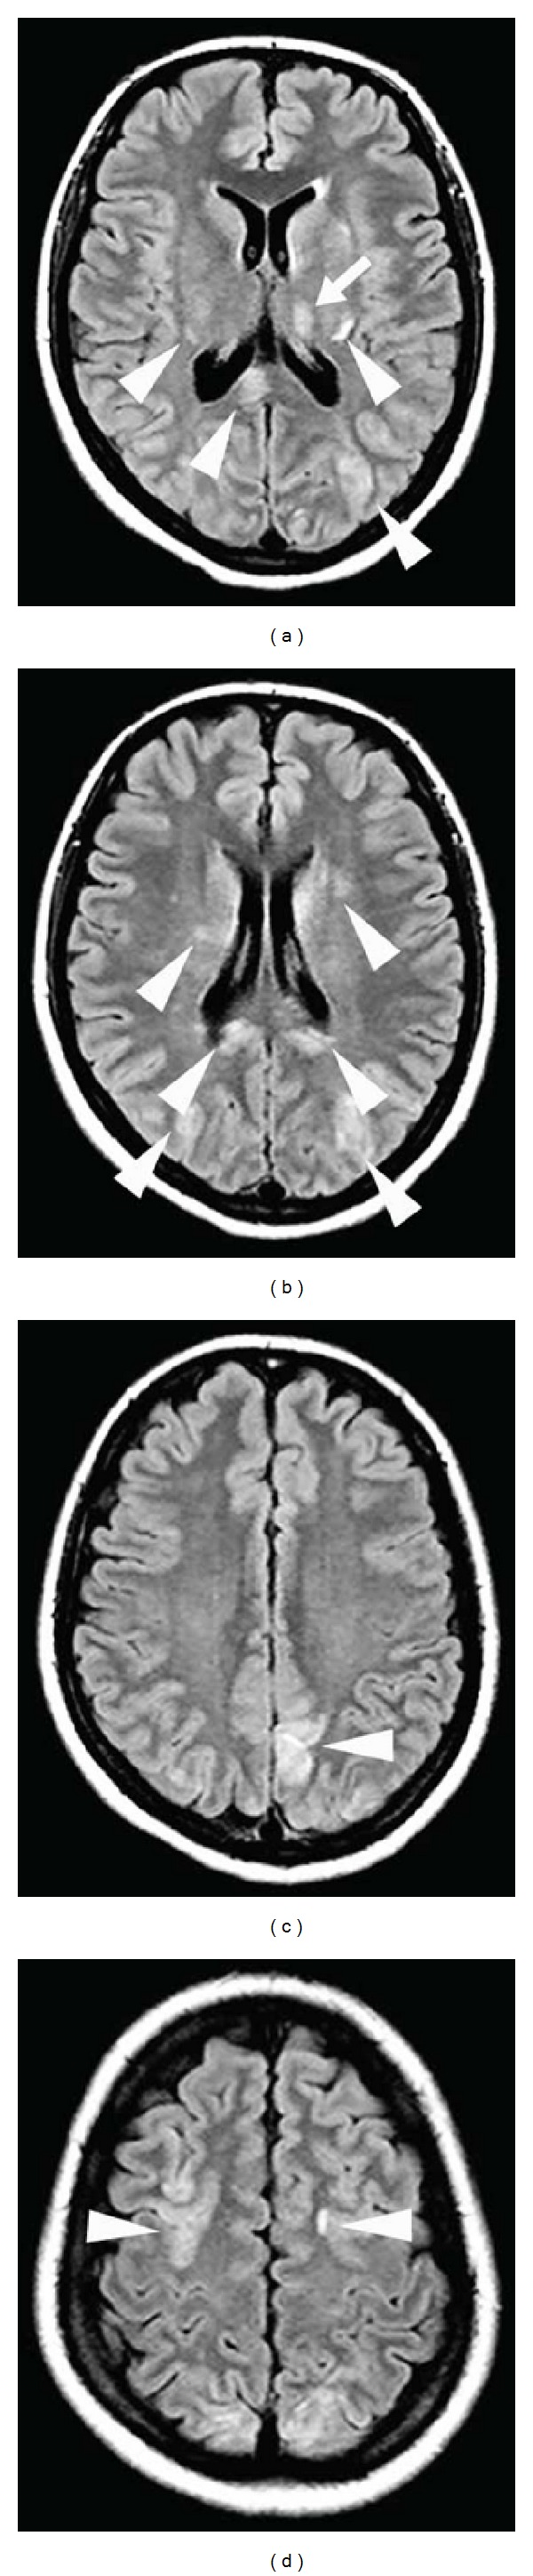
A patient with preeclampsia-related reversible posterior leukoencephalopathy syndrome with cortical and subcortical (arrowhead on a, b, c, and d) vasogenic edema seen as hyperintensity on FLAIR sequences also present in the left thalamus (arrow, a).
